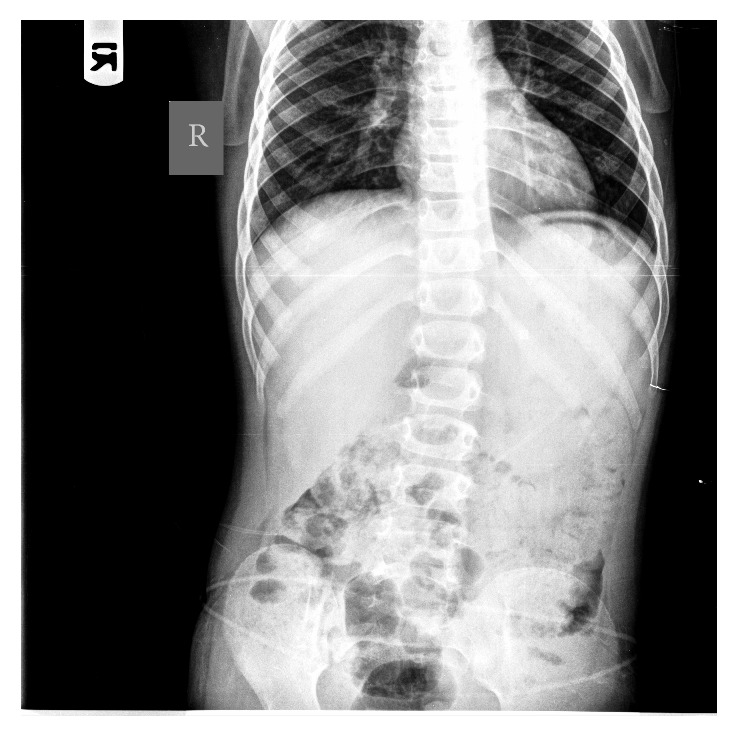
Flat upright X-ray that shows normal chest and scoliosis in thoracolumbar region.
